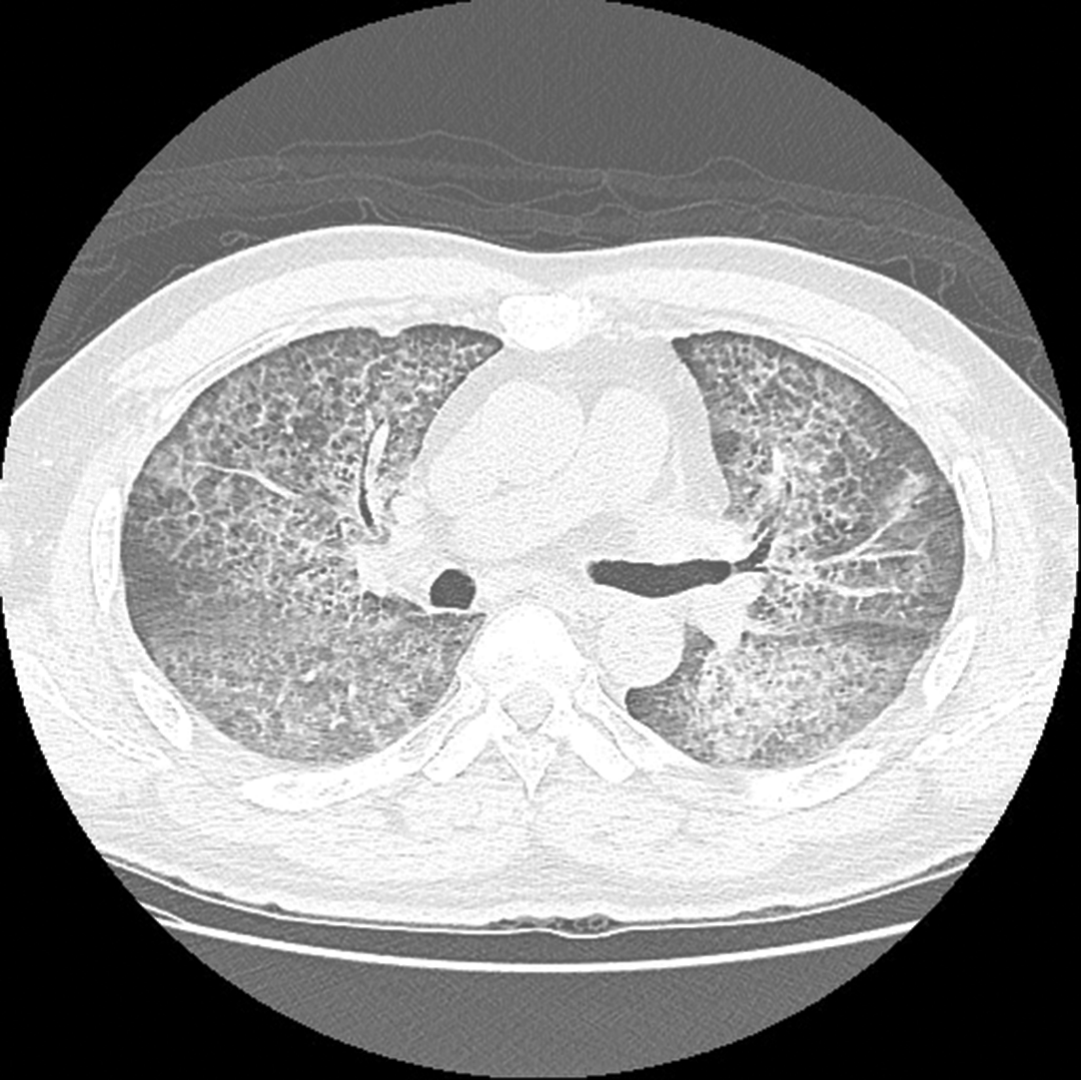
On chest computed tomography (CT), extensive patchy ground‐glass opacities superimposed with thickened interlobular septa and intralobular lines were noted in bilateral lungs, indicating a “crazy paving” pattern.
